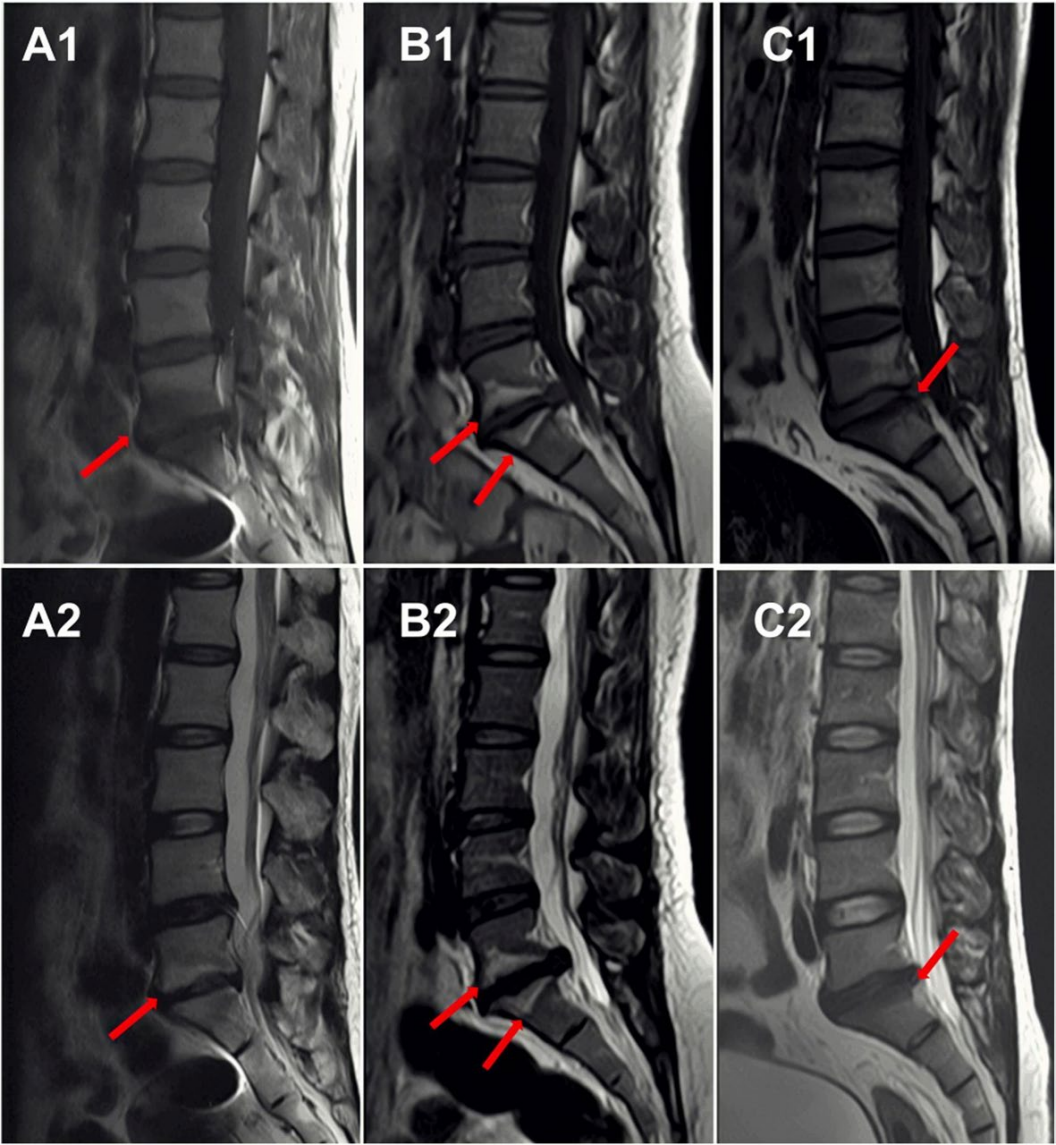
Appearances of three types of MCs. Modic type 1 change (A1, A2): Hypointensity on T1-weighted images (T1WI, A1) and hyperintensity on T2-weighted images (T2WI, A2); Modic type 2 change (B1, B2): Hyperintensity both on T1WI (B1) and T2WI (B2); Modic type 3 change (C1, C2): Hypointensity both on T1WI (C1) and T2WI (C2)
